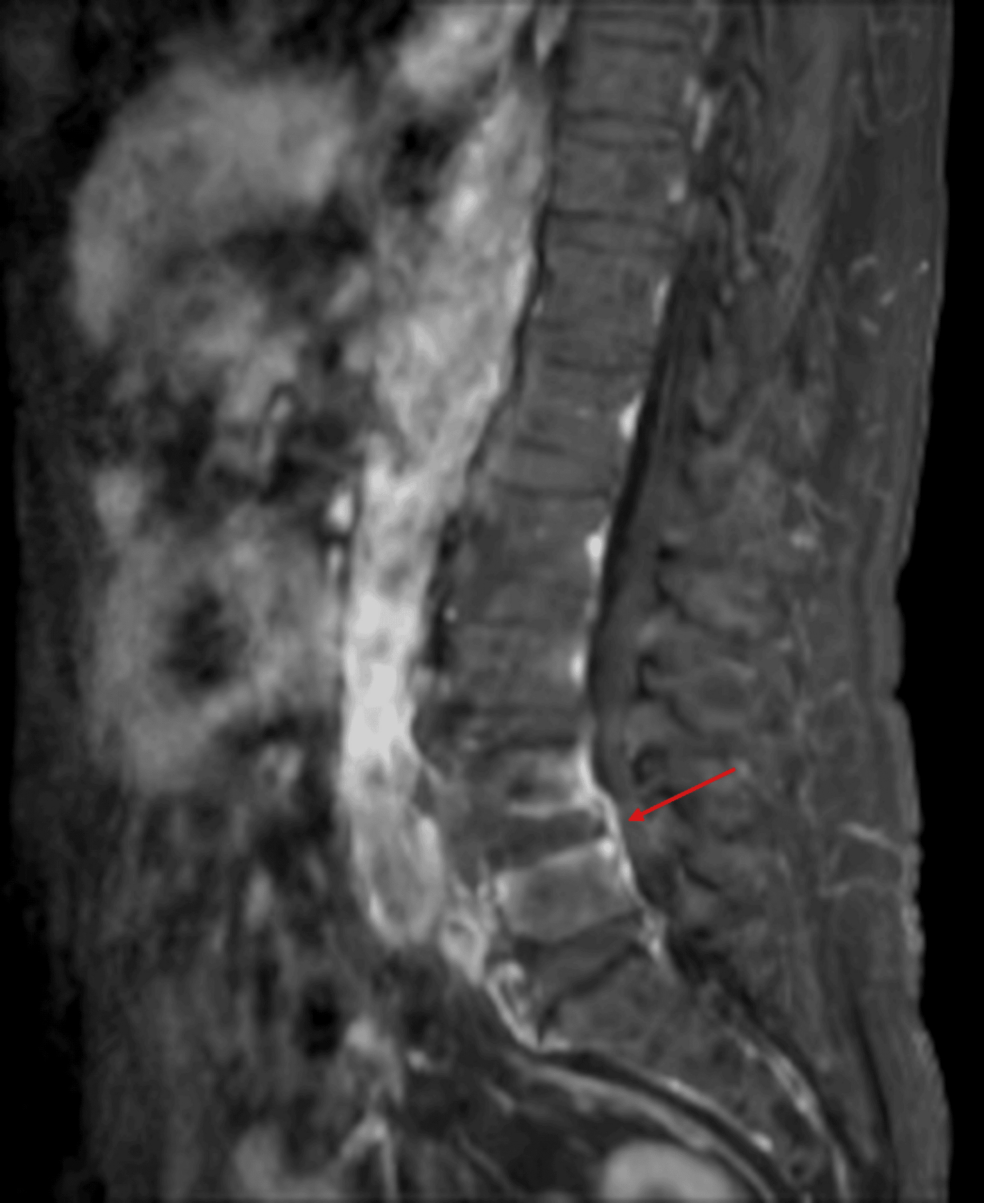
Contrast-enhanced lumbar MRI two weeks post-procedure. The red arrow indicates enhancing soft tissue in the anterior epidural space at L4/5. The red arrow highlights mildly thickened, enhancing soft tissue in the ventral epidural space located just anterior to the dura at the L4/5 level. This faintly brightened area may represent inflammatory, infectious, or reactive changes.
